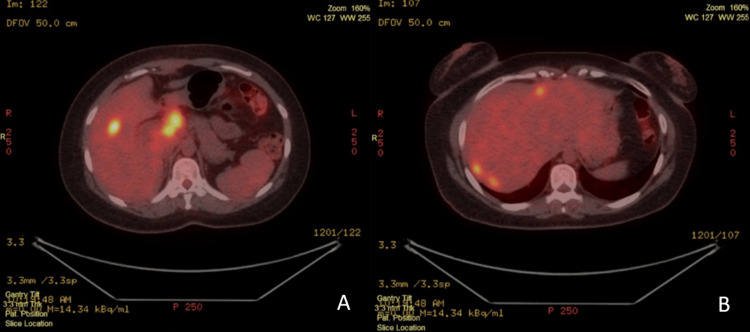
FDG PET/CT demonstrating hepatic and upper abdominal nodal metastases. (A and B) FDG PET/CT scan demonstrating multiple metabolically active metastatic lesions in both hepatic lobes and upper abdominal lymph nodes.
